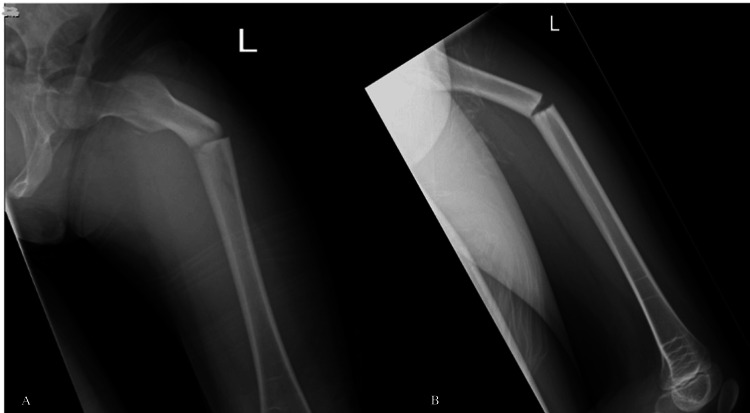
Anteroposterior (A) and lateral (B) radiographs of the left femur demonstrating an atypical diaphyseal femur fracture (Case 1)
